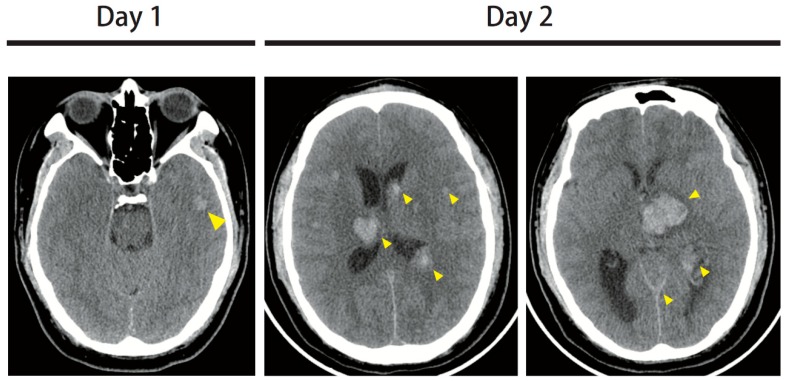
On the day 1 brain computed tomography (CT), a 13 mm lesion with hyperdensity was found in the left temporal region, with suspected intracerebral hemorrhage. On the day 2 brain CT, multifocal intracranial hemorrhages in bilateral cerebral hemispheres were noted, the largest being a 30 mm lesion in the left thalamus. The midline structures were shifted to the right side. Extensive swelling was present in the cerebellum and brain stem.
